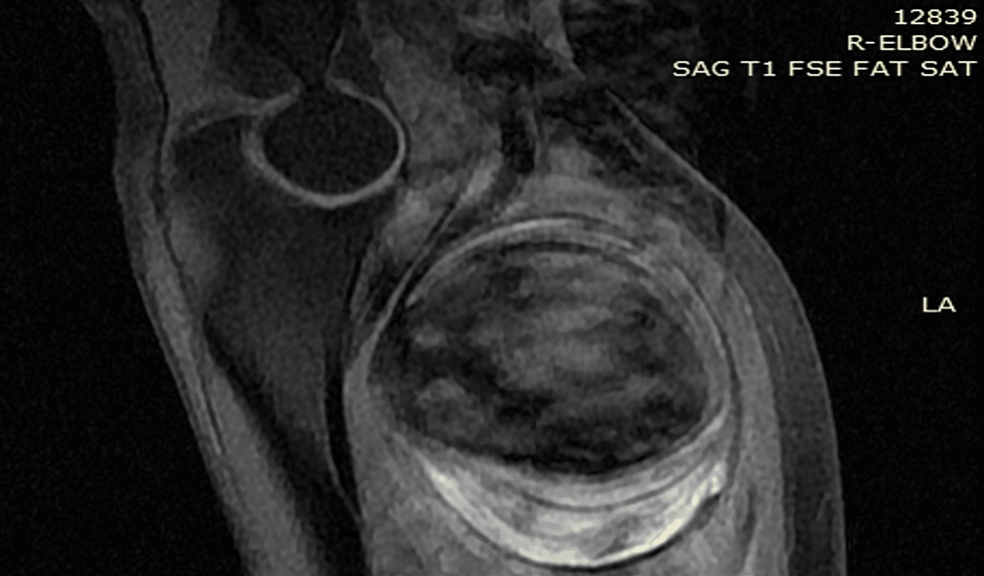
MRI of the right forearm
